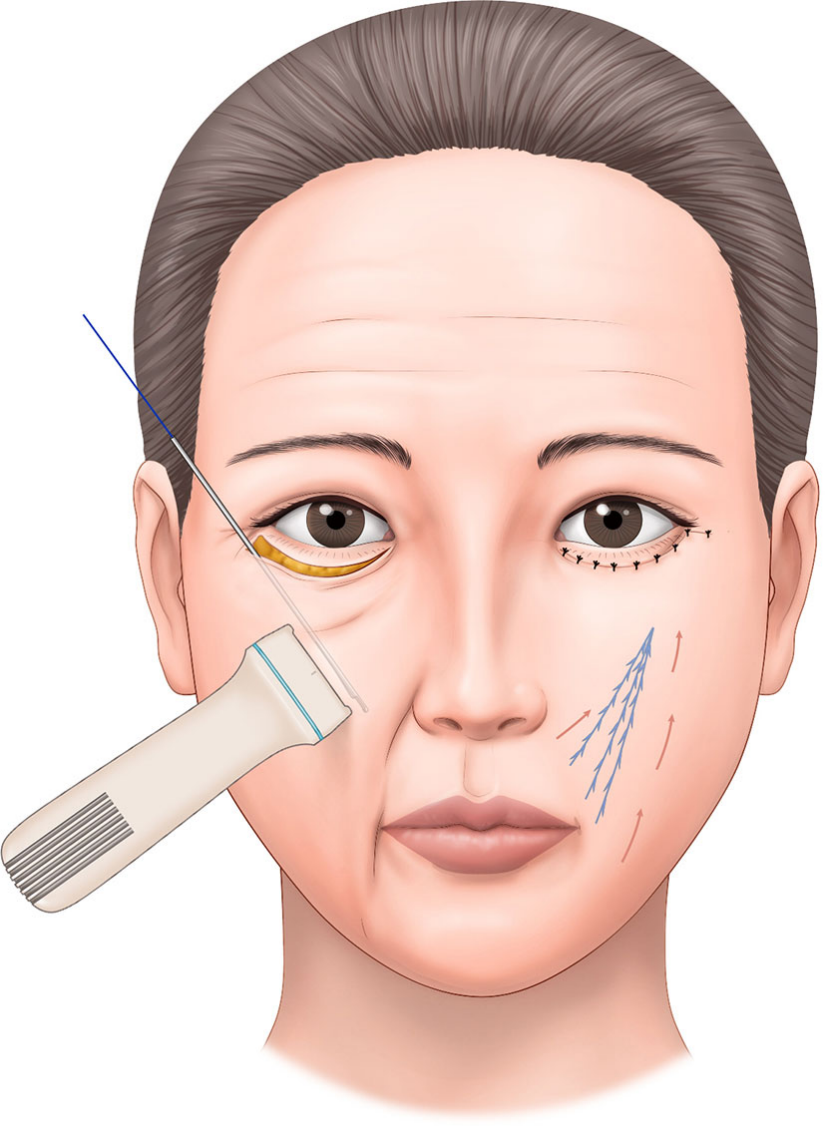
The thread-containing cannula is inserted after passing through the arcus marginalis. The insertion direction is guided towards the superficial layer, traversing the deep fat pad and superficial muscular aponeurotic system (SMAS) layer, and finally stopping superficially under the dermis, medially to the nasolabial fold, while utilizing portable handheld ultrasonography for guidance
